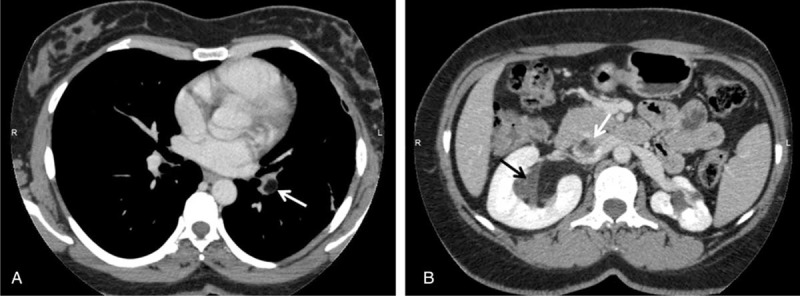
(A) Filling defect (arrow) compatible with fat embolus in the branch of left pulmonary artery is shown on axial CT image. (B) A fat-density lesion in the right renal pelvis (black arrow) extending into the inferior vena cava (white arrow) is demonstrated on axial contrast-enhanced CT image.
